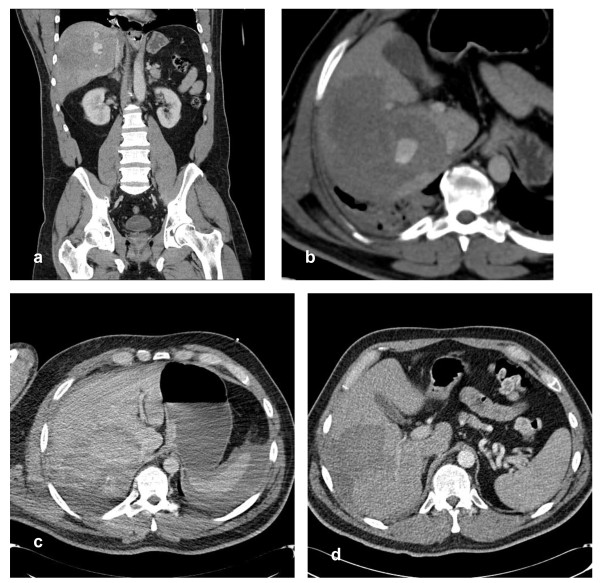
a) Coronal contrast enhanced arterial phase CT reconstruction showing contrast blush in a contained right lobe haematoma due to blunt inury. b) Axial CT demonstrates the blush. c) Scan at 18 hours showing no blush but capsular rupture with intraperitoneal blood. d) Follow up CT at 9 weeks showing resolving right lobe haematoma.
